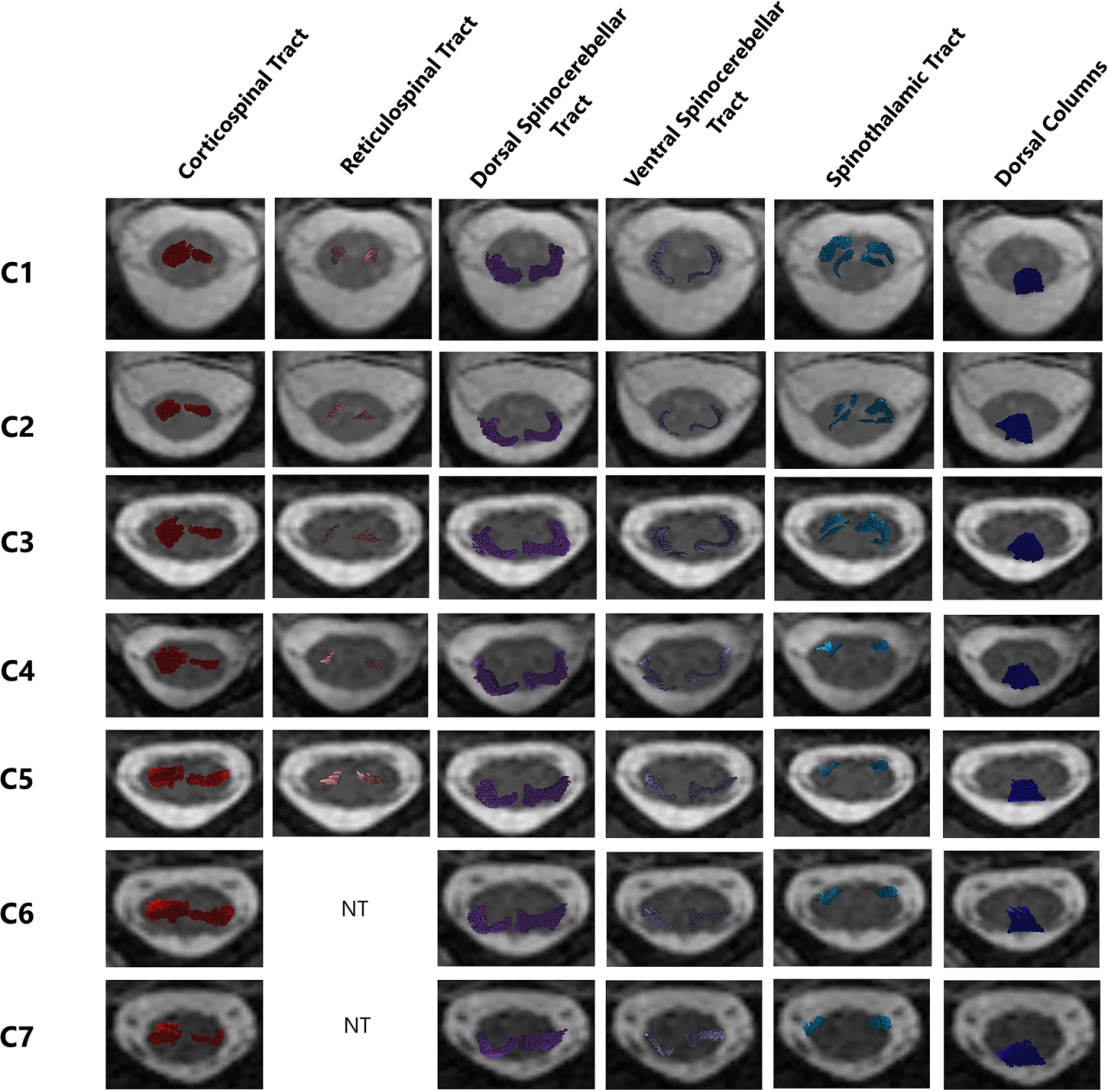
Spinal cord atlas. Each tract is merged on axial T2-weighted images at each cervical spine level. NT, Not tracked
